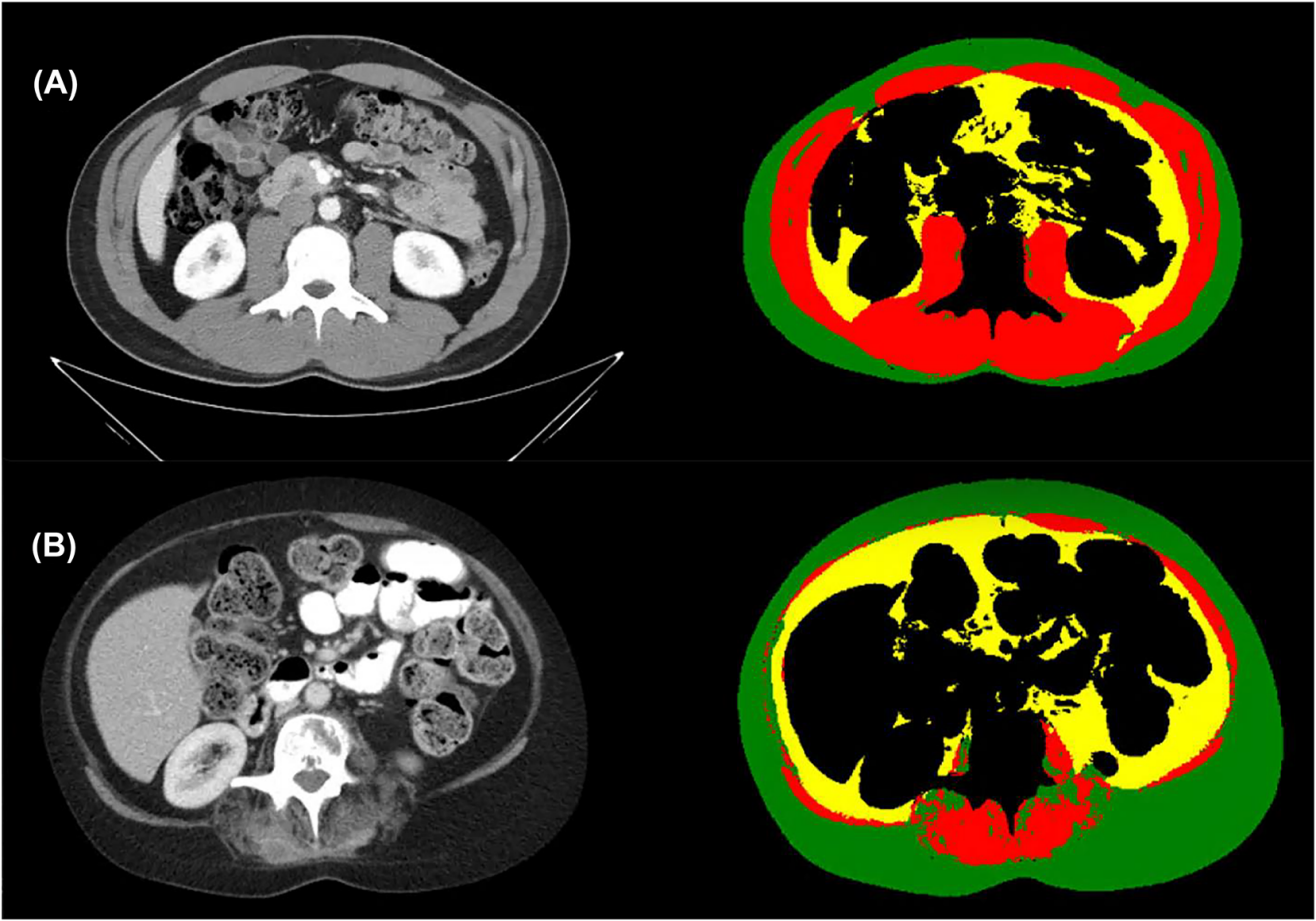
Body composition analysis at the level of the third lumbar vertebral body. Representative axial computed tomography images at the level of the third lumbar vertebral body (left column) and matching segmentation label maps (right column). Patient A was a 26‐ to 30‐year‐old male with a skeletal muscle (red) index (SMI) of 65.9 (4th quartile), a subcutaneous adiposity (green) index (SAI) of 38.6 (2nd quartile) and a visceral adiposity (yellow) index (VAI) of 25.8 (1st quartile). Patient B is a 46‐ to 50‐year‐old female with an SMI (red) of 18.9 (1st quartile), an SAI (green) of 77.3 (2nd quartile) and a VAI (yellow) of 36.92 (3rd quartile).
